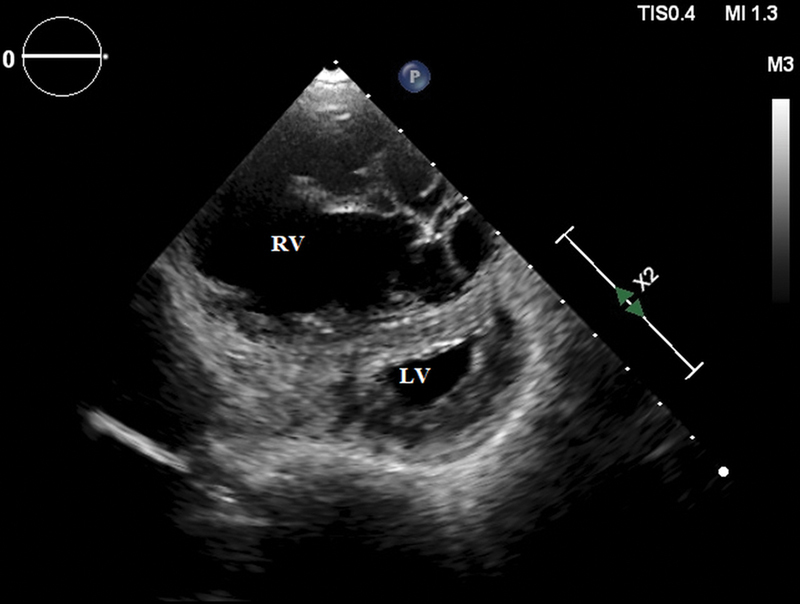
Echocardiogram in parasternal short axis view showing D-shaped LV and dilated RV. LV, left ventricle; RV, right ventricle.
